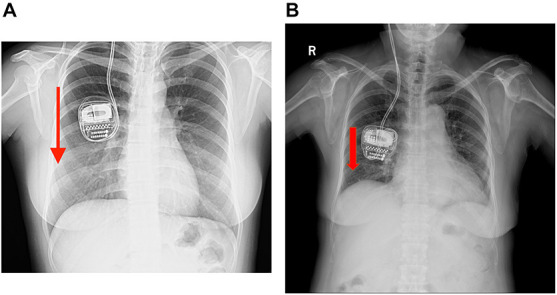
(A,B) Plain chest X-rays of cases 1 and 2 showing the upside-down implanted pulse generators (IPGs) but with the extension wires still intact and no evidence of twisting observed. The red arrow means that the r-IPG was upside down.
